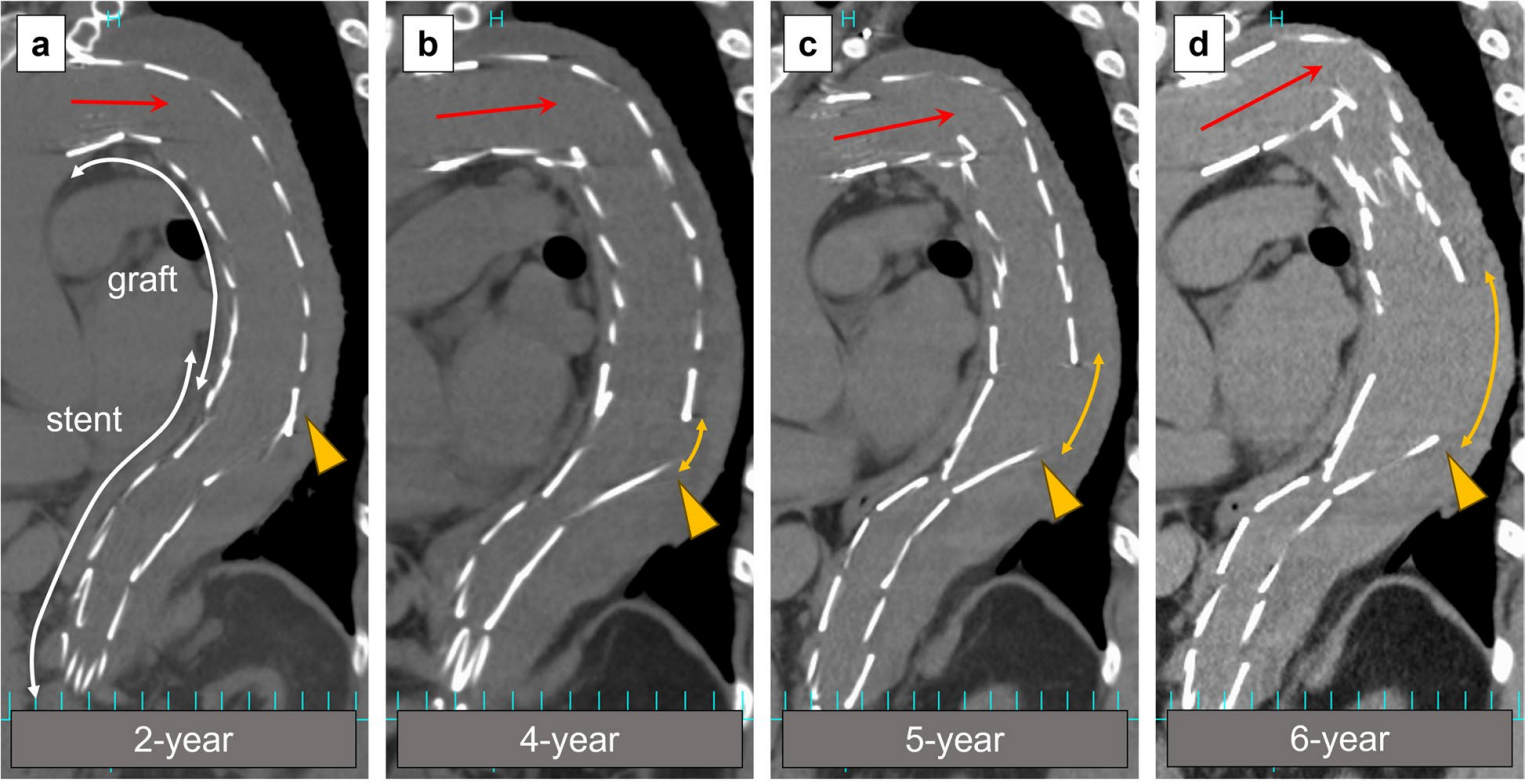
Timeline of device migration. All four panels depict the curved planar reconstruction image generated from the CT scan. These images were obtained at one postoperatively from the first TEVAR. The white line in panel (a) shows the length of the stent-graft and the bare-metal stent. The red arrow represents the direction of the proximal edge of the stent-graft, which moves cranially over time. The yellow arrowhead points to the proximal edge of the bare-metal stent, while the yellow line shows the gap between the stent-graft and the bare-metal stent. The gap was observed at 4 years after surgery and enlarged over time
